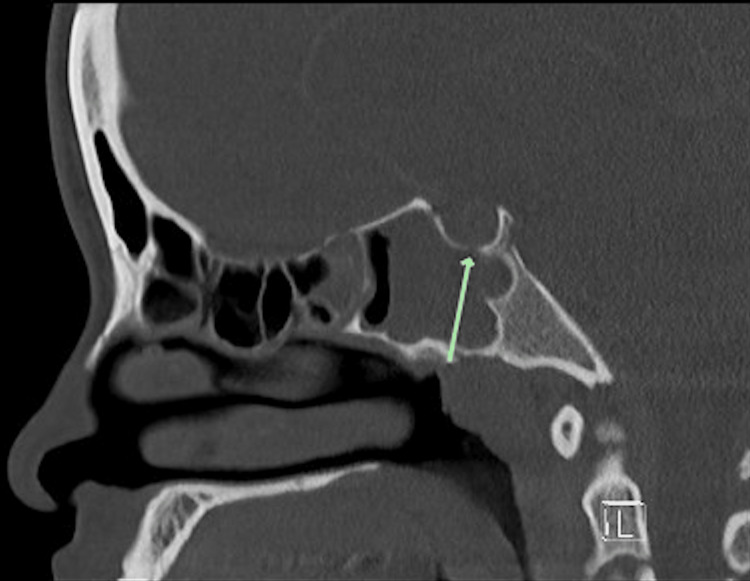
CT of the sinus with contrast, sagittal cut, posterior ethmoid and sphenoid sinus disease with bony dehiscence along superior wall of sphenoid sinus/anterior sella (arrow) CT, computed tomography.
